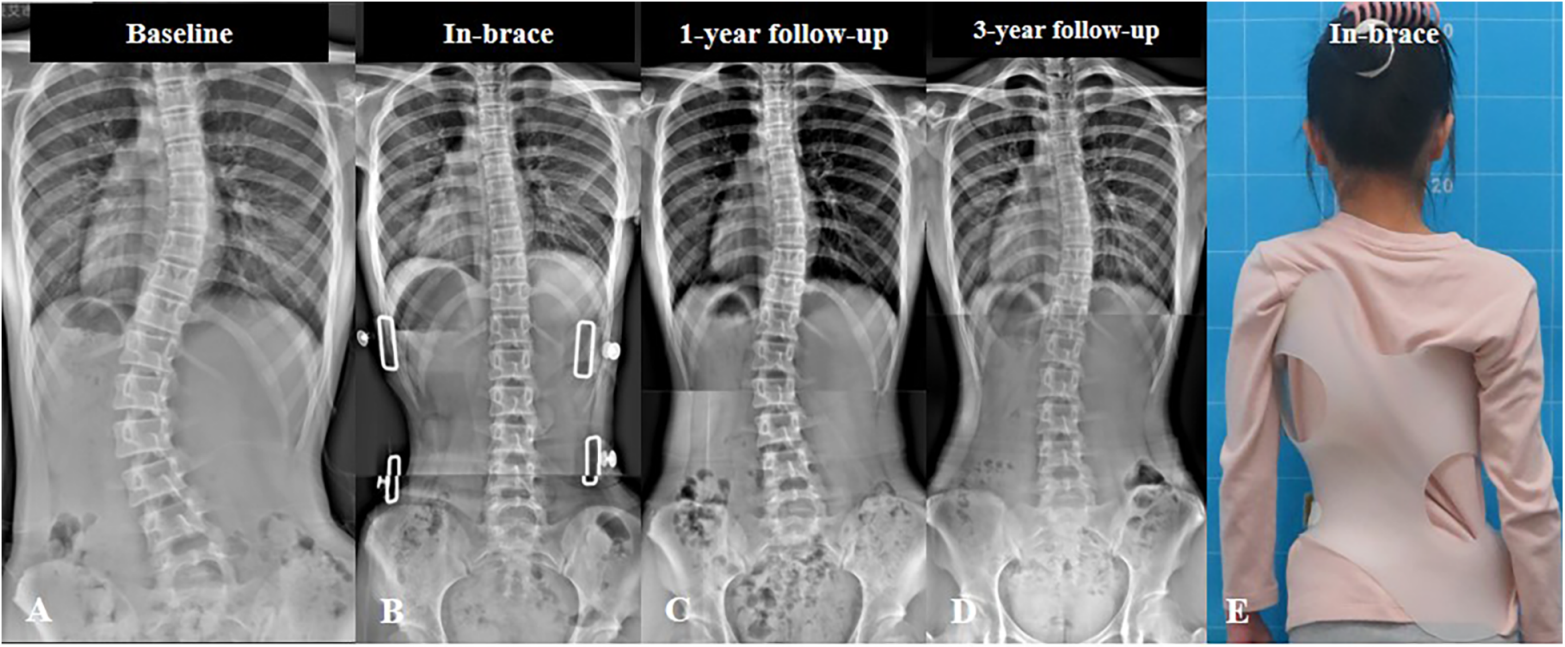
A 13-year-old girl diagnosed with adolescent idiopathic scoliosis treated with thoracolumbosacral orthosis (TLSO). The main lumbar curve was 42°, and the minor thoracic curve was 30° at baseline (A) In-brace radiograph shows excellent correction of both curves (B) After 1 year of treatment, the patient had satisfactory curve improvement (C), which was adequately maintained over a 3-year follow-up period (D) Clinical in-brace appearance of the patient (E).
